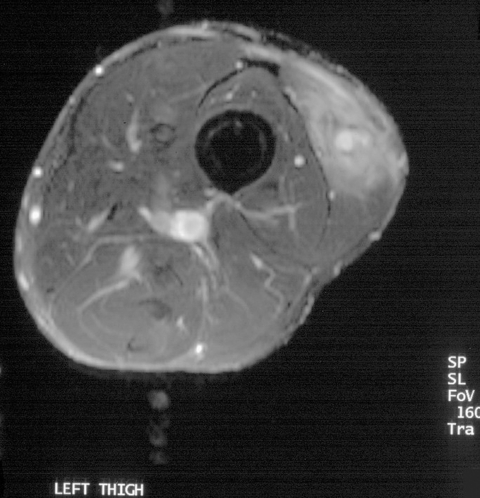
Magnetic resonance image of thigh with *Gnathostoma* larva (case 4).
